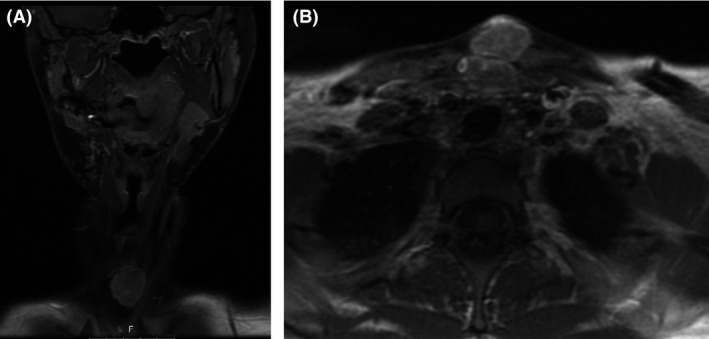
Preoperative coronal (A) and axial (B) gadolinium‐enhanced T1‐weighted MRI neck images of a 21‐year‐old patient with recurrent mandibular osteosarcoma, and iatrogenic osteosarcoma at the tracheotomy tract which was performed as a part of her previous operation.
